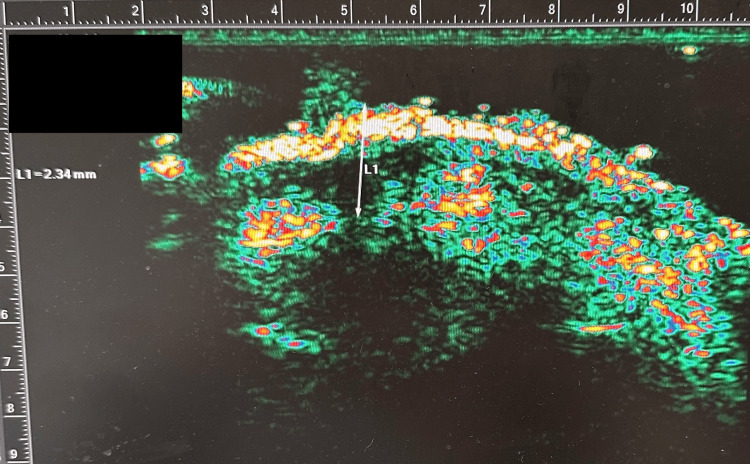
Initial high-resolution dermal ultrasound demonstrating tumor depth prior to treatment. A measured depth of 2.34 mm was within the acceptable treatment threshold of 3 mm. L1 represents the measured depth (2.34 mm) of the tumor prior to radiotherapy treatment.
